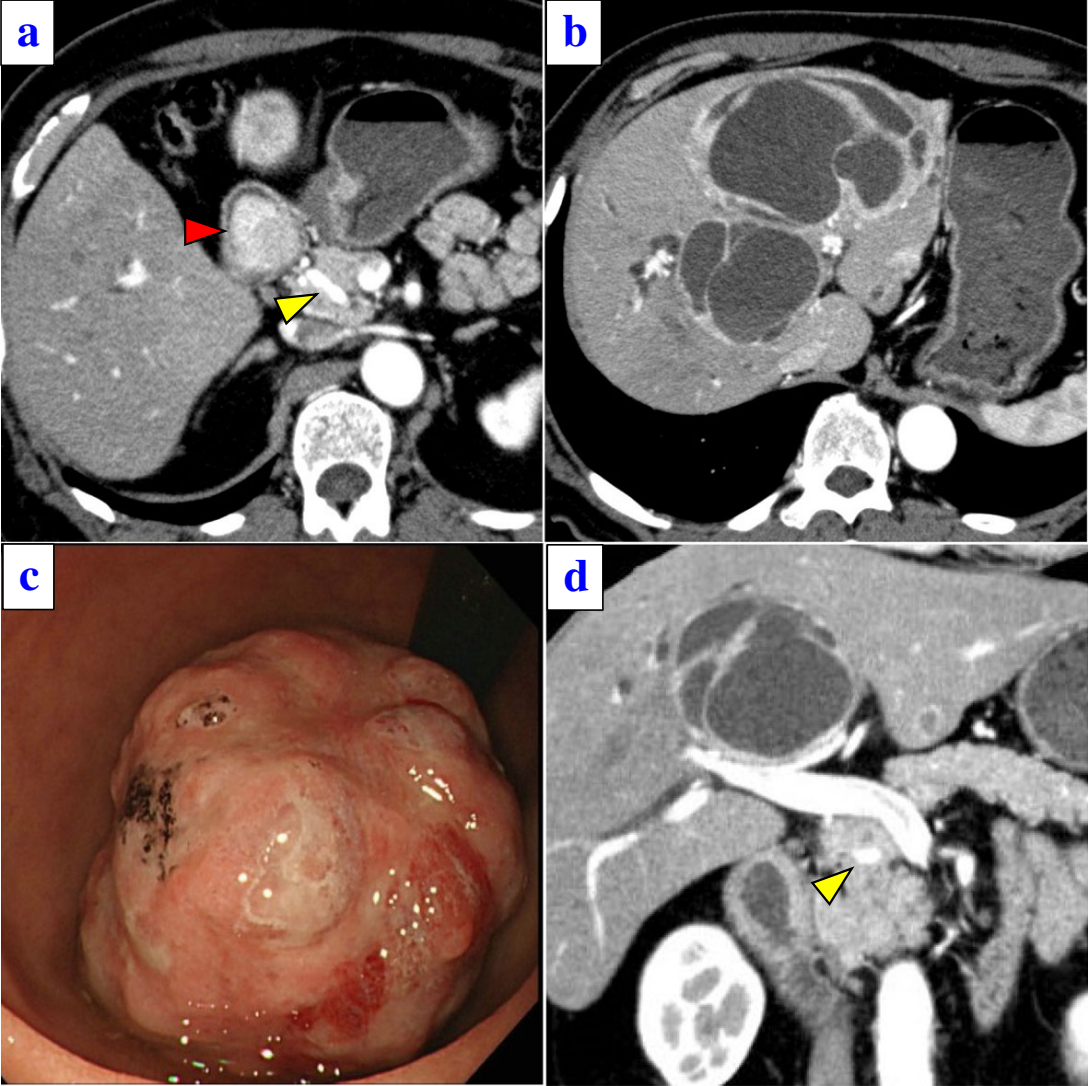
a A duodenal tumor with increased contrast enhancement was observed (red arrow). In addition, the common hepatic artery (CHA) (yellow arrow) branched from the superior mesenteric artery (SMA), passing through the pancreatic head. b Abdominal CT showed multiple metastases in the left three lobes of the liver. c A 3.5-cm-diameter type 1 tumor in the descending portion of the duodenum was revealed by gastroscopy. d A coronal image. The yellow arrow is the CHA branching from the SMA, passing through the pancreatic head
